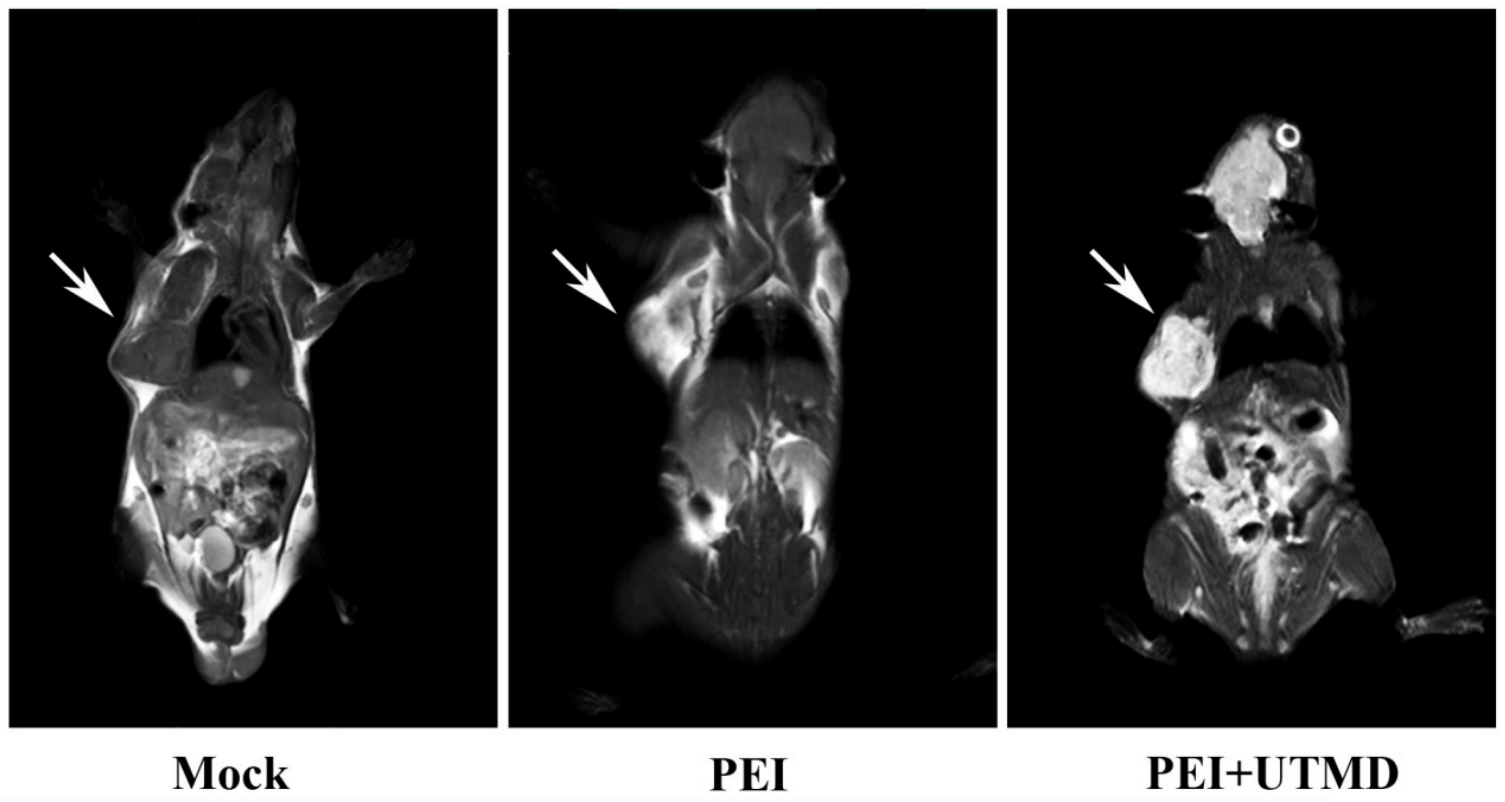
Polyethyleneimine (PEI) nanohydrogels have the potential for tumor magnetic resonance imaging (MRI).
